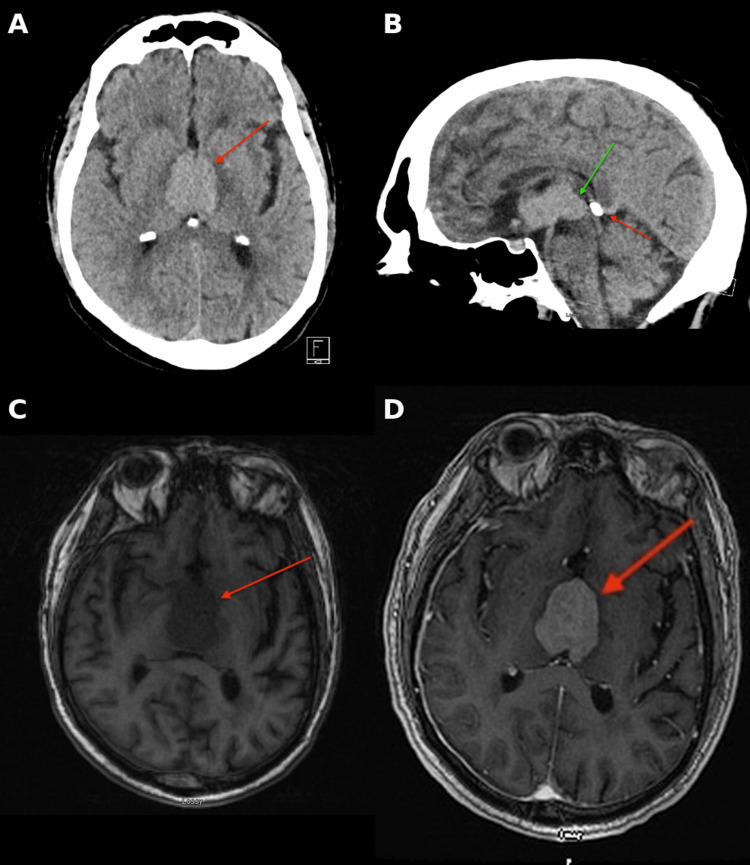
Multimodal neuroimaging demonstrating pineal-region lymphoma (A) Axial noncontrast CT demonstrating a pineal-region mass (red arrow) anterior to the calcified pineal gland with partial effacement of the posterior third ventricle. (B) Sagittal noncontrast CT showing the soft-tissue density mass (green arrow) anterior to the calcified pineal gland (red arrow), delineating its craniocaudal extent. (C) Axial T1-weighted MRI without contrast demonstrating the midline pineal-region lesion (red arrow), biopsy-proven high-grade B-cell lymphoma. (D) Axial fluid-attenuated inversion recovery MRI post-contrast confirming the centrally located tumor (red arrow) within the posterior third ventricle. The deep midline location adjacent to the posterior third ventricle is clinically relevant because local mass effect and associated neuroendocrine pathway disruption may contribute to inappropriate antidiuretic hormone secretion.
